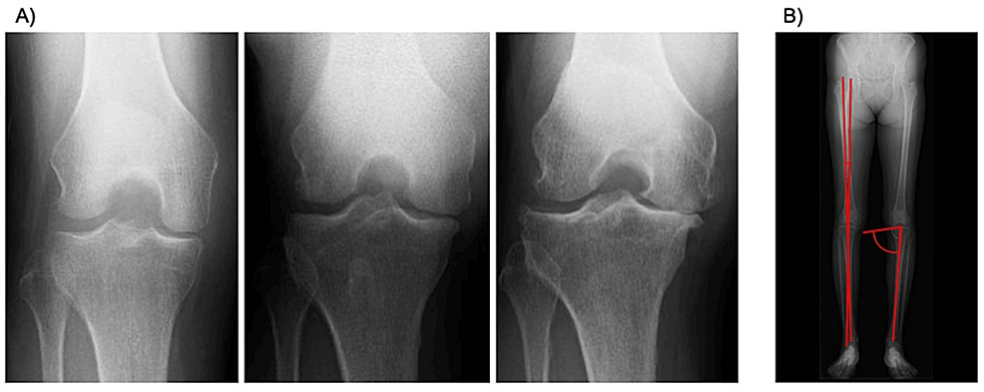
Imaging data analysis with plain X-ray pictures. A) Representative images of the anteroposterior view of the knee joint for Grades 2, 3, and 4 evaluated with Kellgren-Lawrence classification. B) Measurement of the HKA angle and MPTA referring to an anteroposterior full-length lower limb plain X-ray image. The HKA angle is determined by measuring the angle between the line connecting the center of the femoral head and the center of the distal femoral condyle and the line connecting the center of the tibial articular surface and the ankle joint center. MPTA is determined by measuring the angle between the line connecting the medial and lateral edges of the tibial articular surface and the line connecting the center of the tibial articular surface and the ankle joint center. HKA angle: Hip-knee-ankle angle, MPTA: Medial proximal tibia angle
